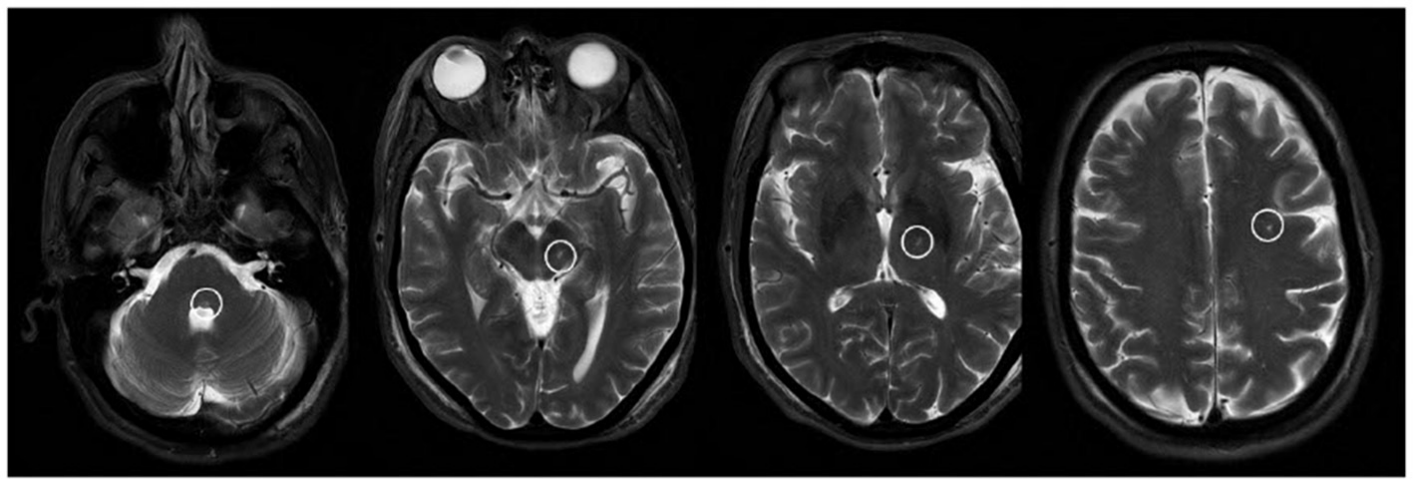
T2-weighted MR images showing the cannula tract as a T2 hyperintensity extending from the dorsal pons through the midbrain, thalamus, and subcortical white matter.
